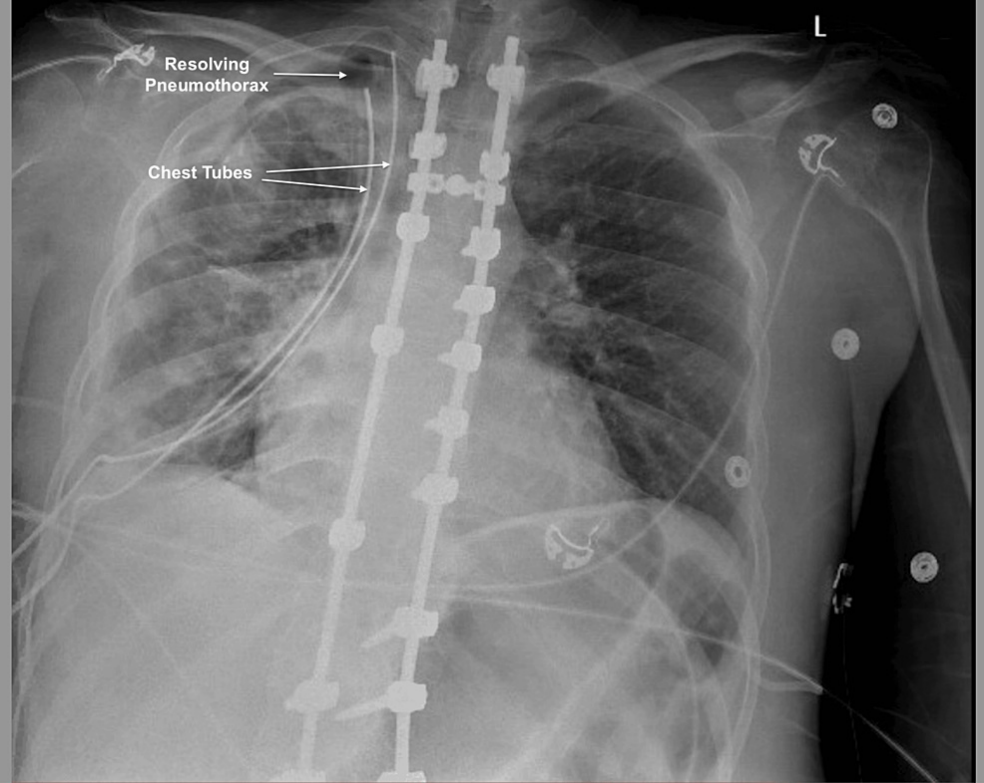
Chest X-ray after chest tube placement with resolving pneumothorax
